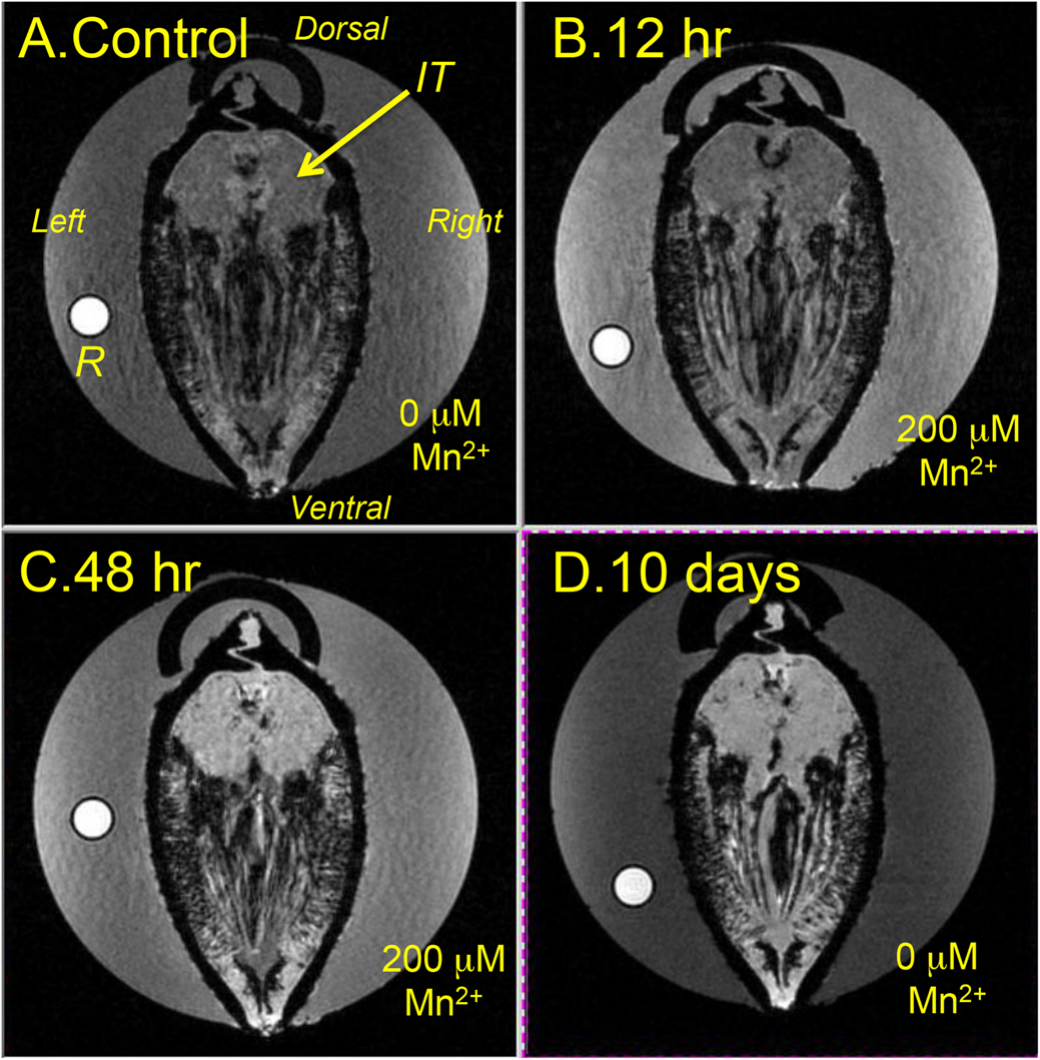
Manganese uptake of the kidneys of *N. douglasiae.* (A) Transverse *T*_1w_-MR image of a living mussel at the posterior side of the kidney corresponding to the IT before the addition of manganese ion. (B) Transverse *T*_1w_-MR image after 12 h (B), 48 h (C) and 10 days (D) of incubation in a pure water containing 200 µM Mn^2+^ at 20°C. Image intensity was measured compared with that of reference capillary (R) containing 0.5 mM of MnCl_2_.
